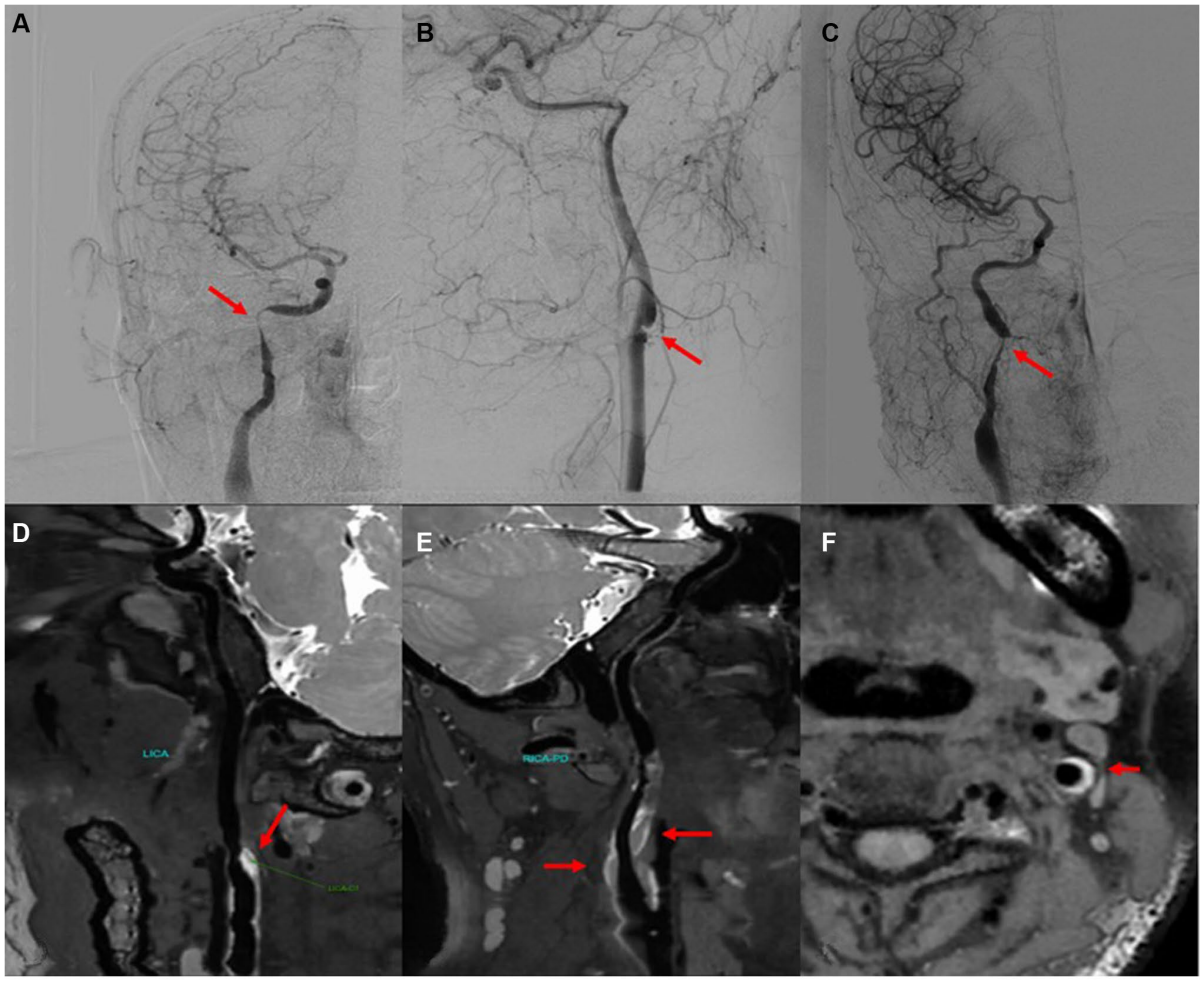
CeAD imaging (arrow). (A–C) Digital subtraction angiography showing vascular dissection. (D,E) High resolution magnetic resonance demonstrating vascular lumen wall hematoma (white high signal). (F) Axial MRI scan showing a mural hematoma and residual lumen.
